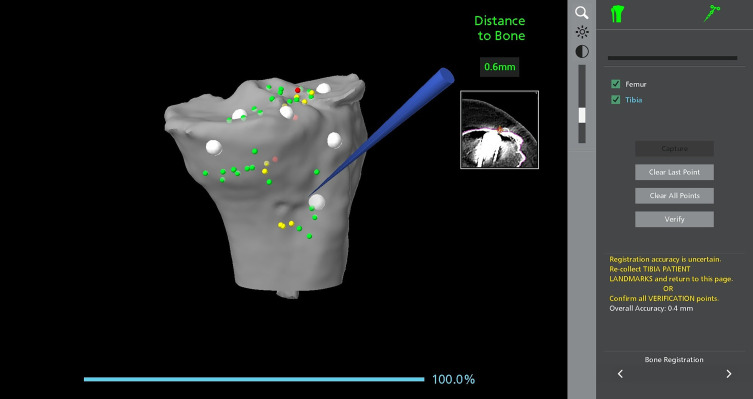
Intraoperative identification of the tibial fixation staple using the image-based robotic system. The robotic probe is utilized to locate the fixation staple, which was entirely covered by bone and not visible through direct visualization. The system provides real-time spatial feedback, displaying the distance to the bone and mapping reference points for accurate positioning. The inset image on the right shows the corresponding CT scan with the staple’s location, ensuring precise intraoperative identification.
